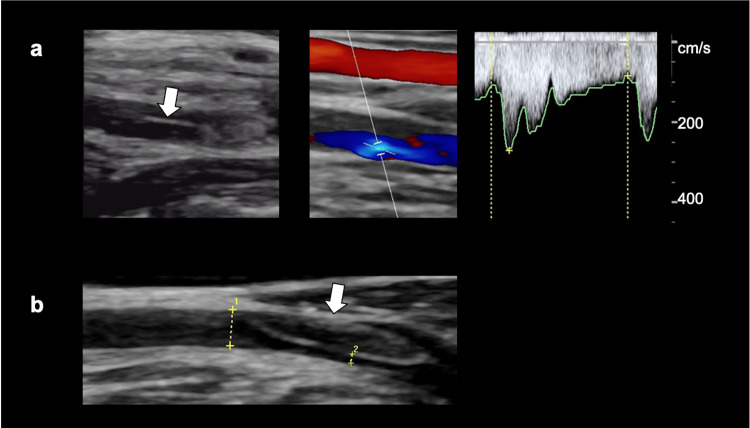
Carotid artery ultrasound a. The right vertebral artery showed a flap in the proximal V1 segment (white arrow) and a mosaic flow pattern with stenosis in the distal V1 segment (peak systolic velocity: 260 cm/s); b. The left vertebral artery showed a thrombosed false lumen near its origin (white arrow).
